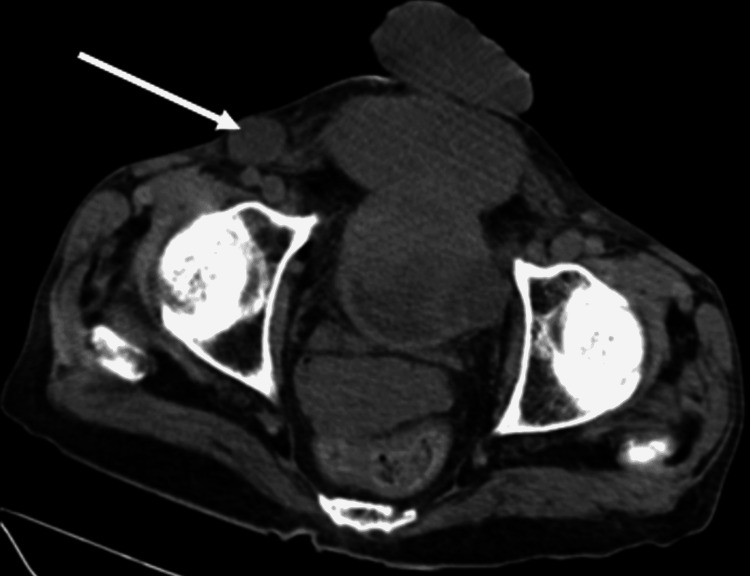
Non-contrast computed tomography scan showing right inguinal lymphadenopathy of largest size measuring 2.7 x 1.6 cm.
